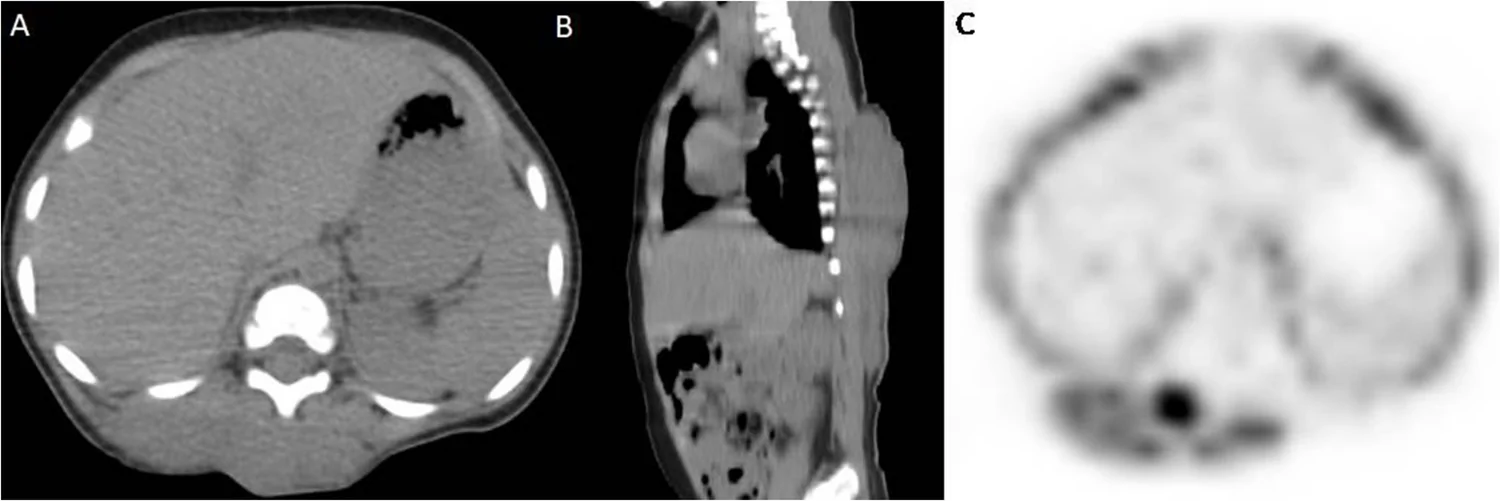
Non-contrast axial (A) and sagittal (B) computed tomogram component of positron emission tomography (PET) image shows a lobulated soft tissue mass in the back crossing the midline and involving the paraspinous muscles. It shows uptake on the PET image (C)
